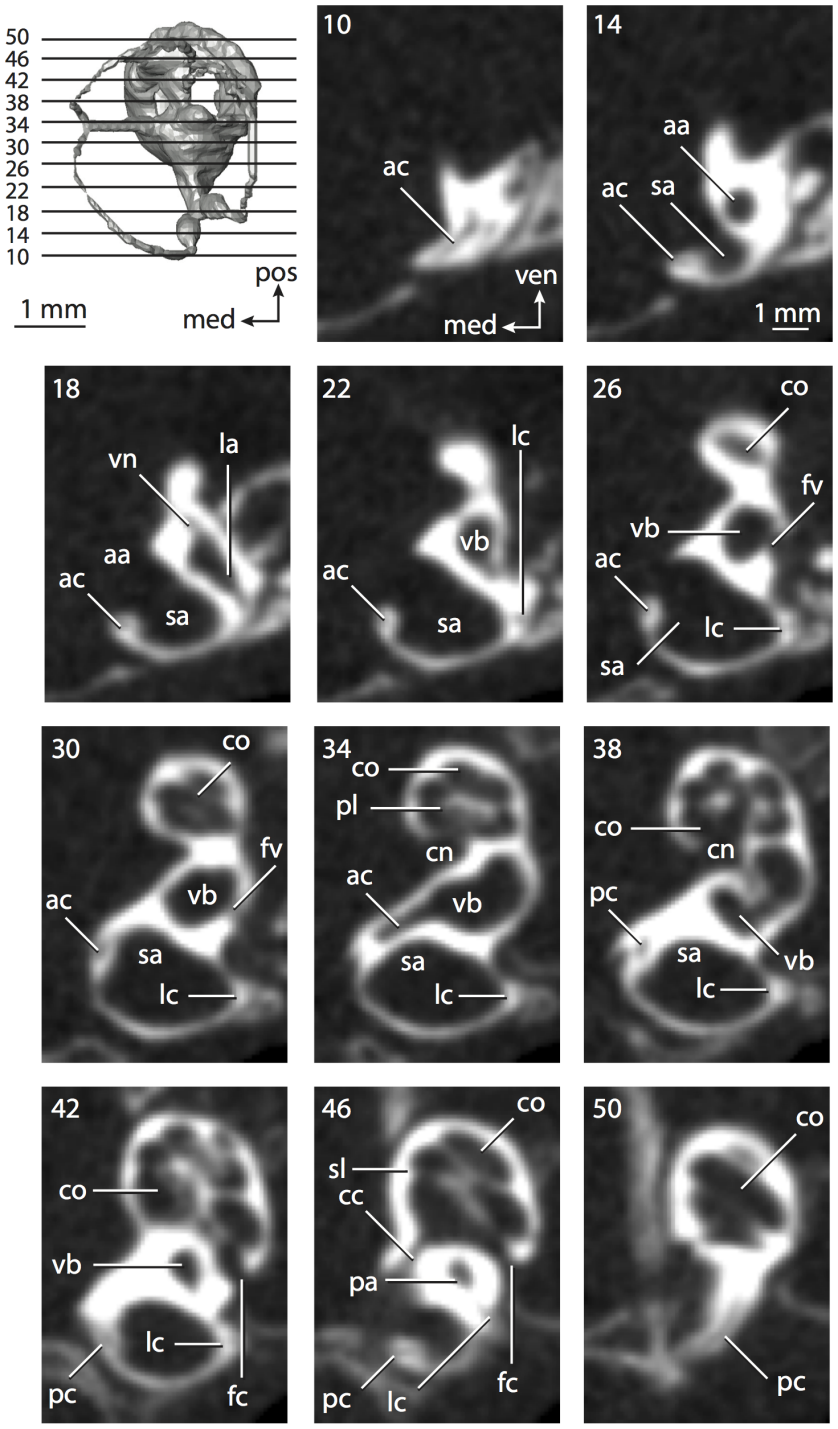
CT slices through ear region of*Nycteris grandis*. Abbreviations listed at the end of the Materials and Methods section.
